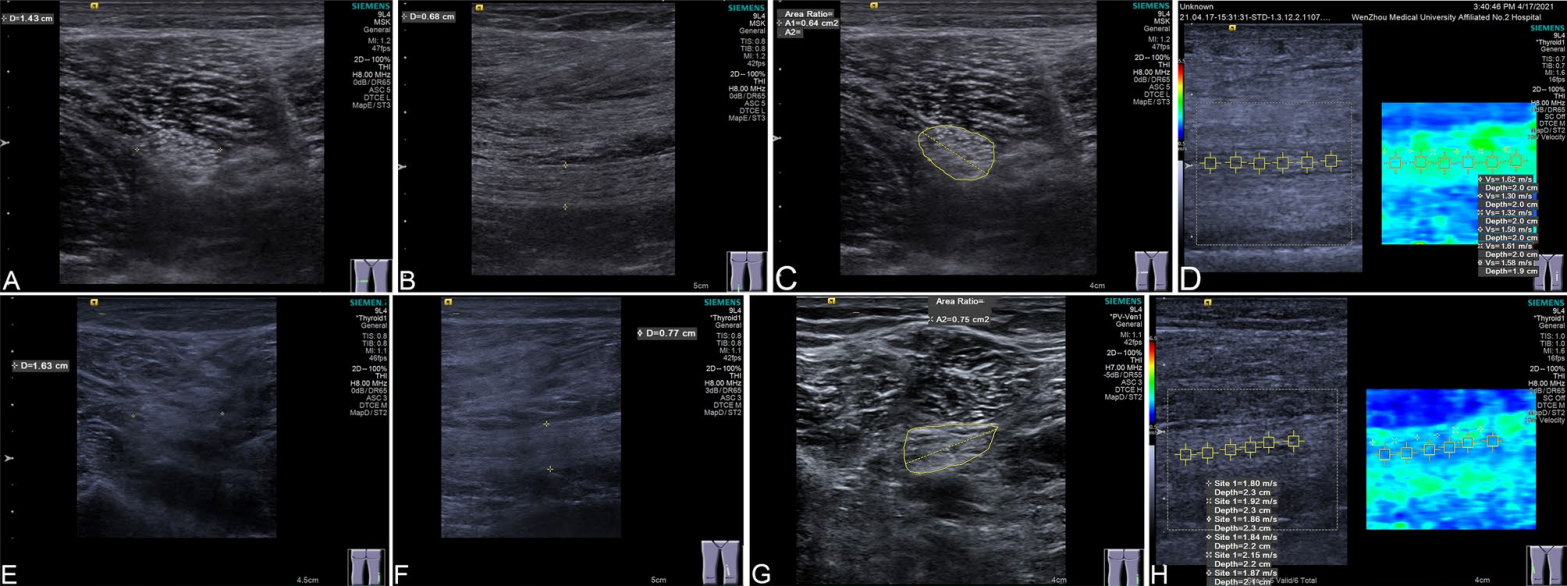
Representative figures of color Doppler ultrasound with a VTIQ quantitative analysis system. The cross-sectional area (CSA) of sciatic nerve was measured by Trace method. The shear wave velocity (SWV) was detected by VTIQ technique. (A) Width of sciatic nerve in T2DM patients without DPN. The largest diameter is 1.43 cm and presented by yellow crossed-stripe symbol. (B) Thickness of sciatic nerve in T2DM patients without DPN. The largest diameter is 0.68 cm and presented by yellow crossed-stripe symbol. (C) CAS of T2DM patient was marked by a yellow circle (the area = 0.64 m^2^). The dotted line indicated the largest diameter. (D) SWV of T2DM patient was ranged from 1.30 to1.62 m/s. (E) Width of sciatic nerve in DPN patient. The largest diameter is 1.63 cm and presented by yellow crossed-stripe symbol. (F) Thickness of sciatic nerve in DPN patient. The largest diameter is 0.77 cm and presented by yellow crossed-stripe symbol. (G) CAS of DPN patient was marked by a yellow circle (the area = 0.75 m^2^). The dotted line indicated the largest diameter. (H) SWV of DPN patient was ranged from 1.80 to 2.15 m/s
